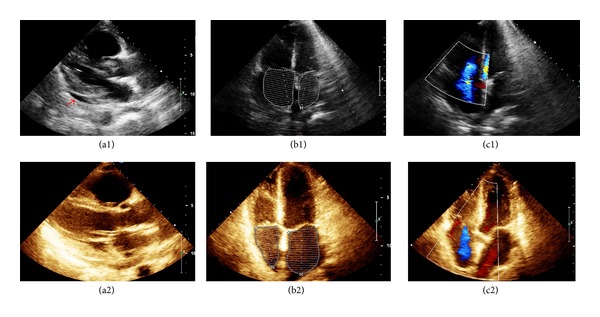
Transthoracic echocardiogram. (a1) Long parasternal axis showing pericardial effusion (arrow); (a2) one week later showing resolution of pericardial effusion; (b1) four-chamber view showing marked right atrial dilatation; (b2) four-chamber view one week later showing normalization of right atrial size; (c1) color doppler showing moderate tricuspid regurgitation; (c2) color doppler one week later showing a decrease in tricuspid regurgitation.
